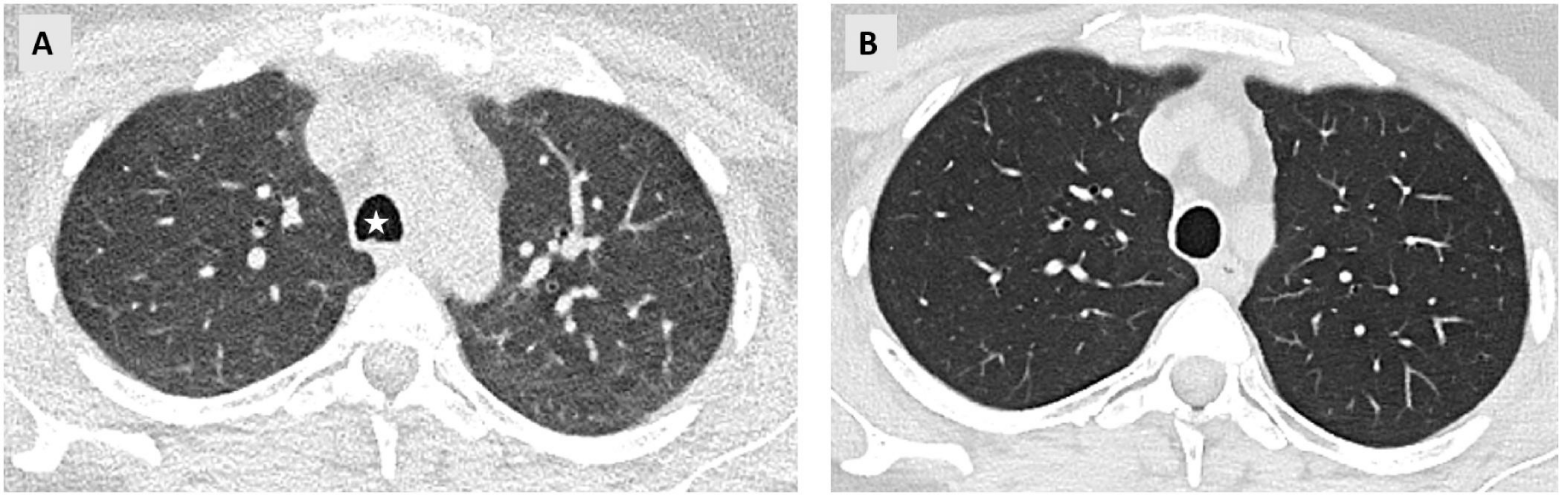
(A) Axial CT scan through the upper lobes showing diffuse ground-glass opacities. The posterior wall of the trachea shows a concave configuration (star) due to an expiratory scan. (B) Inspiratory CT scan with a rounded tracheal configuration showing well aerated lung parenchyma with no ground-glass opacities.
